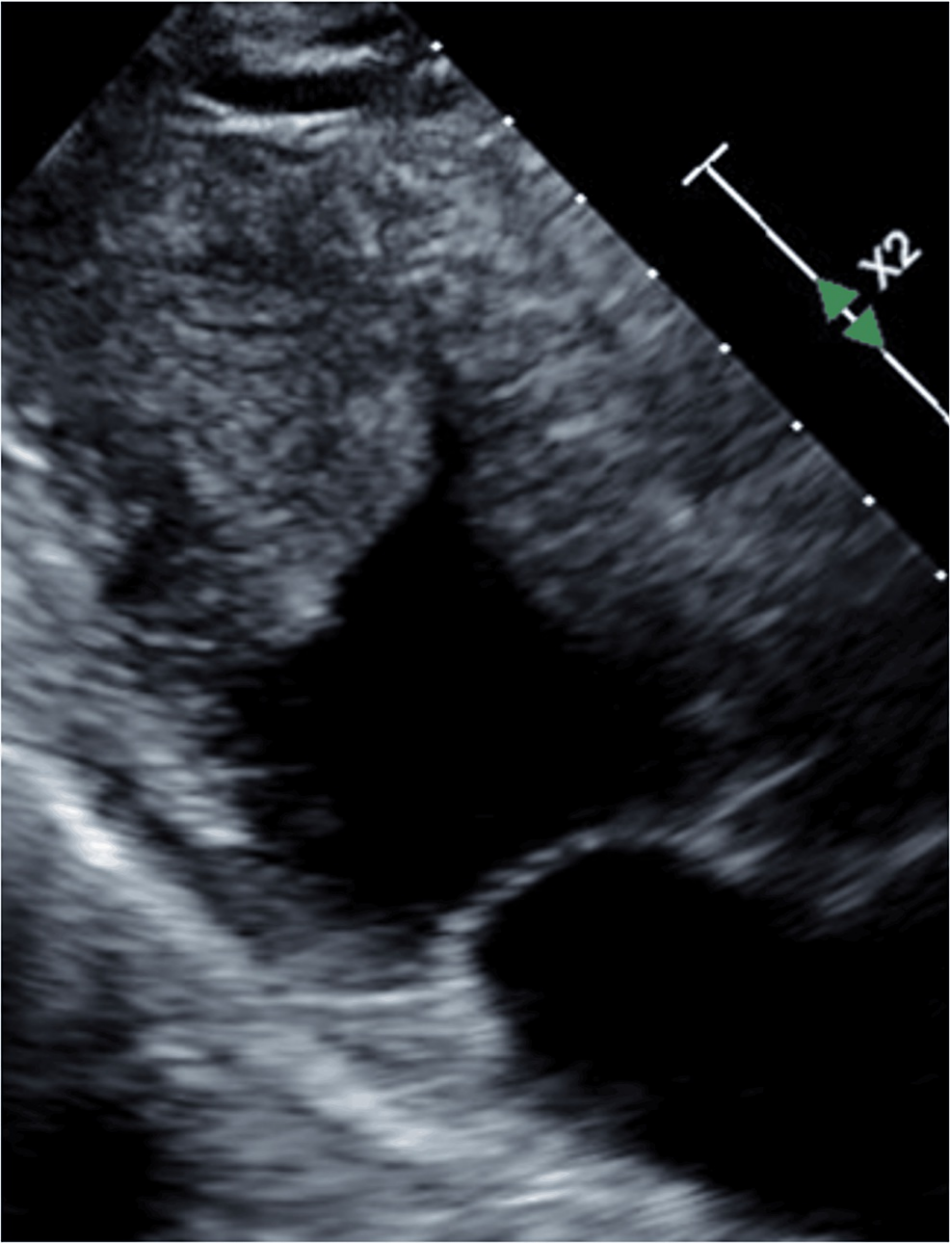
Echocardiographic findings Echocardiography revealed a mobile mass in the left ventricular apex.
